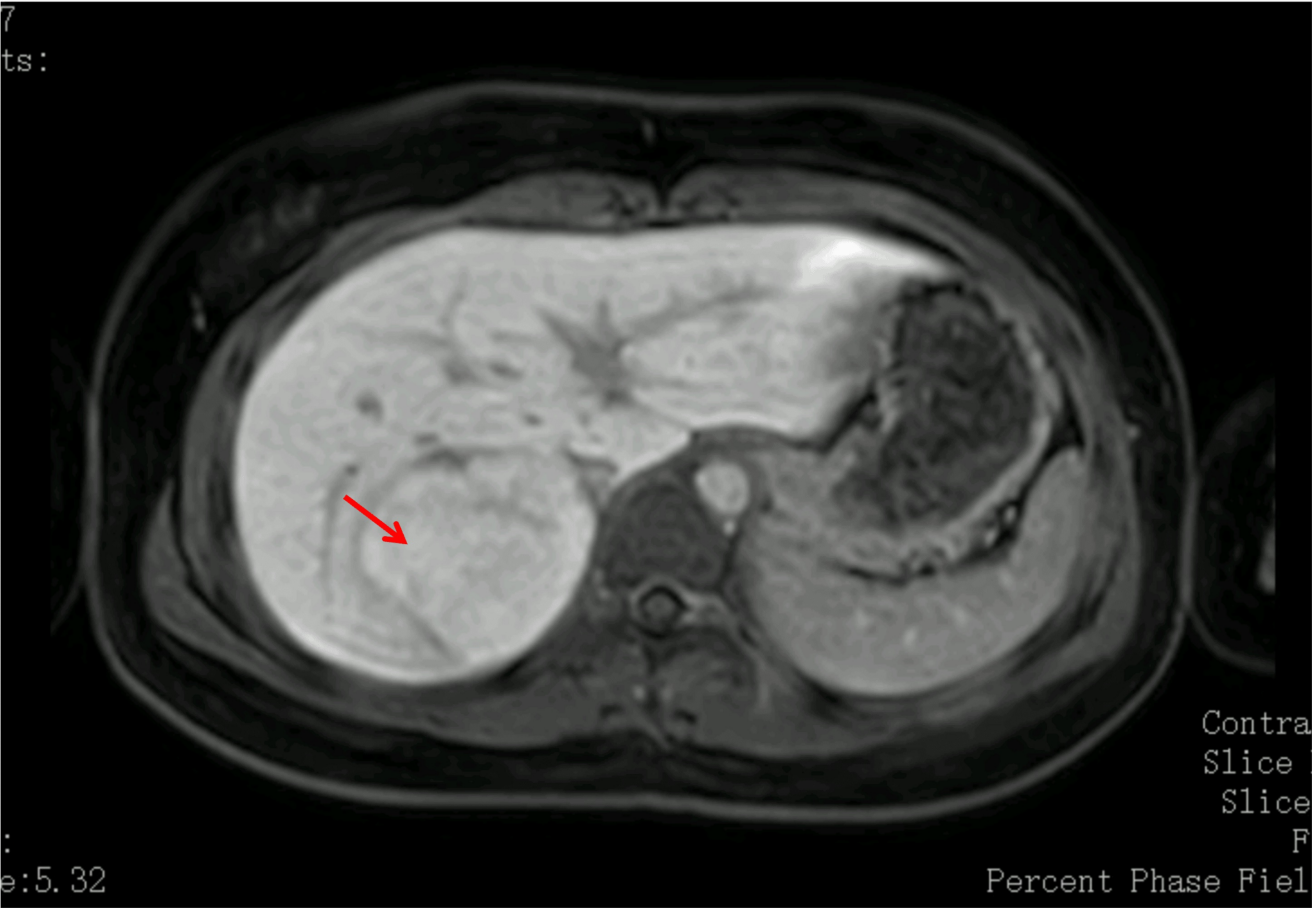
Abdominal MRI with hepatocyte-specific contrast showing a lesion isointense to the surrounding liver parenchyma (arrow), consistent with FNH, with no central scar MRI: magnetic resonance imaging; FNH: focal nodular hyperplasia
